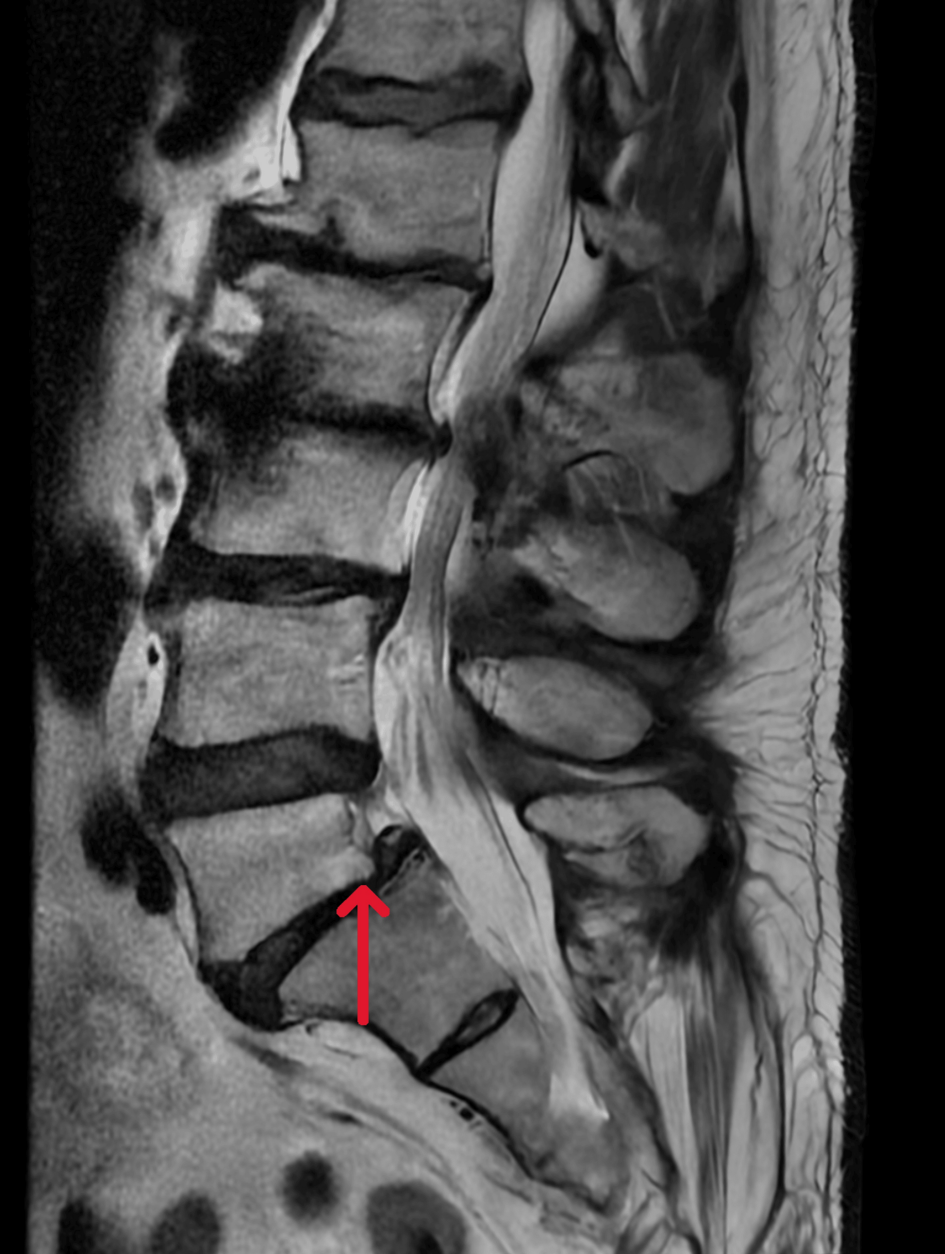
Lumbar MRI Lumbar MRI (T2-FSE, sagittal plane) showing L5 anterolisthesis.
